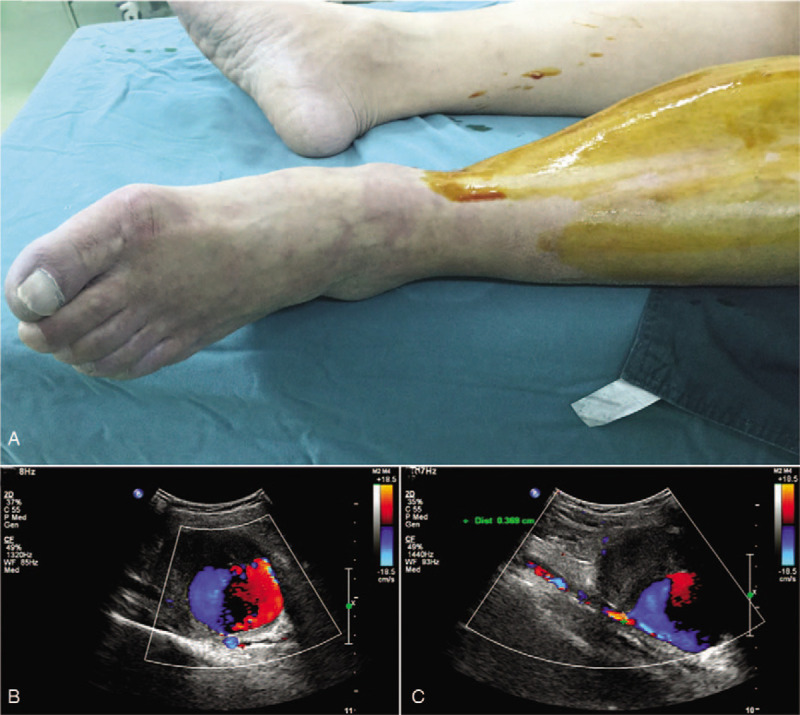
(A) Picture of the preoperative ischemic left shank and foot. (B) Ultrasonographic image of the bloodstream within PSA and (C) the neck and lateral thrombus of PSA. PSA = pseudoaneurysm.
